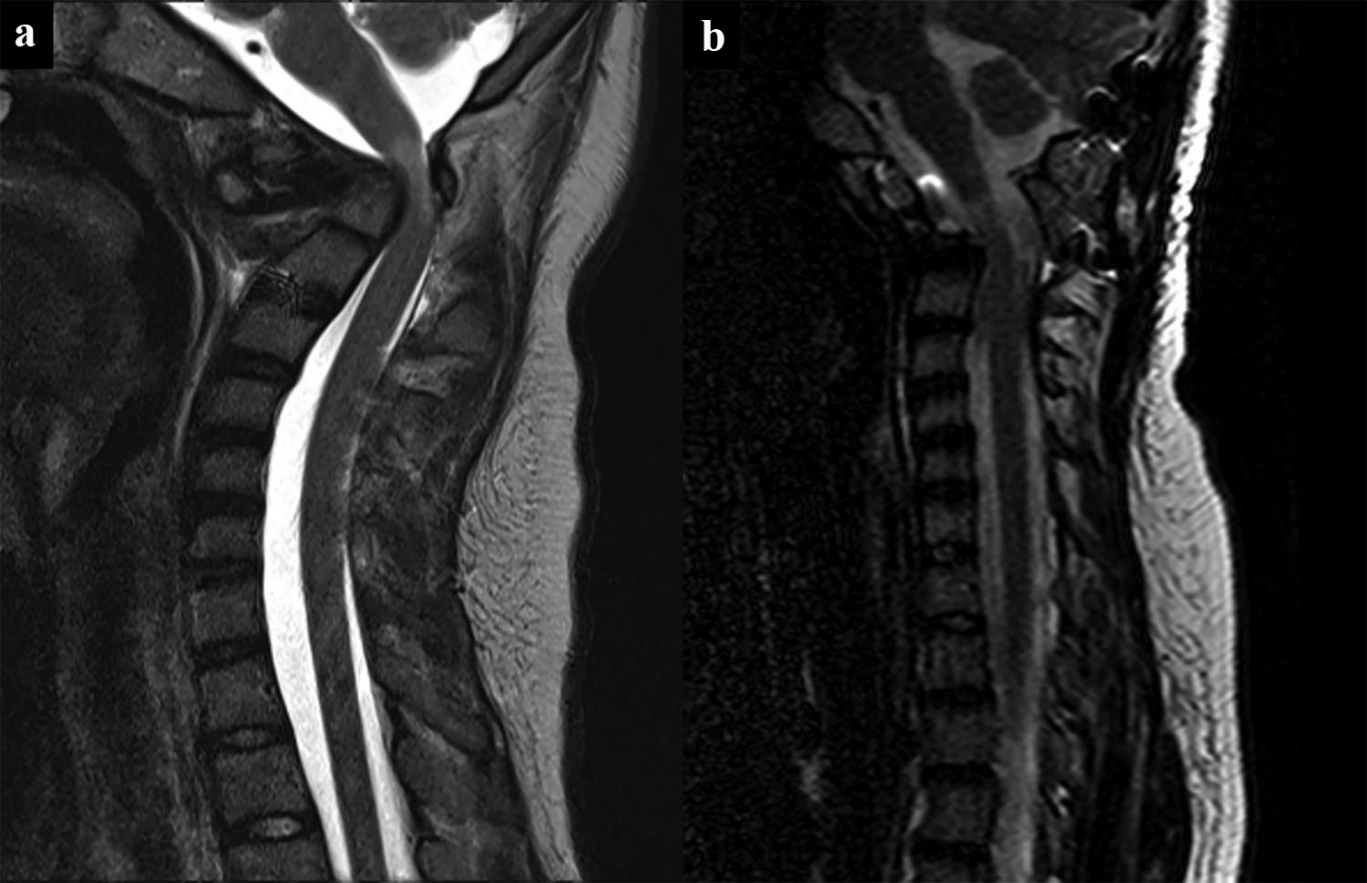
Use of Halo for safe intraoperative flipping. a Preoperative sagittal T2-weighted MRI shows compression with myelopathy. This patient was placed in Halo fixation intraoperatively for the flip. b Postoperative sagittal T2-weighted MRI 3 years after transoral odontoidectomy and O-C fusion with TLS shows reduction of compression
